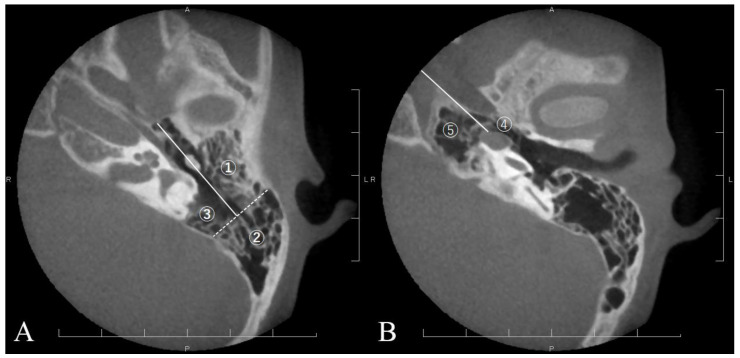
Axial CT images in the left temporal bone, indicating five regions of pneumatized air cell systems by the white line (A): level of the atticoantrum, showing the anterolateral area of periantral cells (①), posterolateral area of periantral cells (②), and medial area of periantral cells (③). The white solid line indicates the longitudinal axis of the atticoantral cavity. The white-dashed line is orthogonal to the longitudinal line at posterior end of mastoid antrum. (B) Level of the tympanic cavity, showing peritubal cells (④) and petrous apex cells (⑤). The white solid line connecting the inside of the carotid artery with otic capsule divides the two areas.
